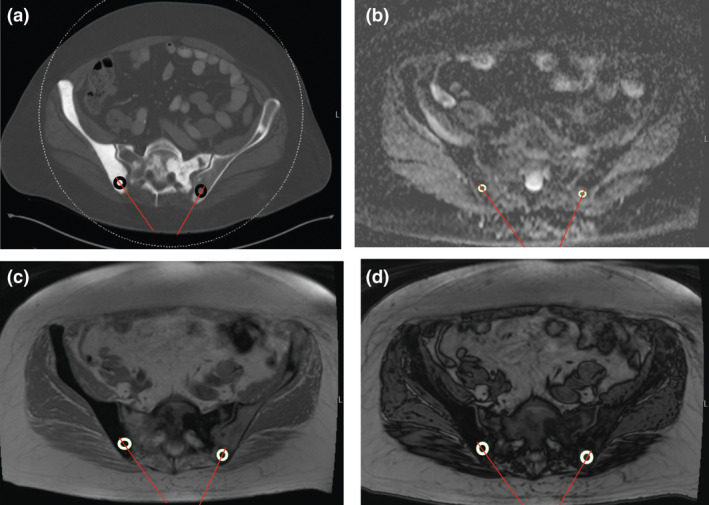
(A) Measurement of mean CT attenuation of lesions in the right and left posterior ilium in a 75‐year‐old man with suspected castrate‐resistant metastatic prostate cancer. The lesion in the right ilium had a CT attenuation of 1063 HU. The lesion in the left posterior ilium had a CT attenuation of 387 HU. Red lines show the biopsy needle paths. (B) Measurement of the signal intensity of lesions in the right and left posterior ilium on ADC MRI sequence in a 75‐year‐old man with suspected castrate‐resistant metastatic prostate cancer. The lesion in the right ilium had a mean ADC of 175 mm^2^/s and the lesion in the left posterior ilium had a mean ADC of 921 mm^2^/s. Red lines show the biopsy needle paths. (C) Measurement of the signal intensity of lesions in the right and left posterior ilium on in‐phase MRI in a 75‐year‐old man with suspected castrate‐resistant metastatic prostate cancer. The lesion in the right ilium had a mean signal intensity of 9.86 and the lesion in the left posterior ilium had a mean signal intensity of 41.46. Red lines show the biopsy needle paths. (D) Measurement of the signal intensity of lesions in the right and left posterior ilium on out‐of‐phase MRI sequence in a 75‐year‐old man with suspected castrate‐resistant metastatic prostate cancer. The lesion in the right ilium had a mean signal intensity of 6.42 (SIR 0.65) and the lesion in the left posterior ilium had a mean signal intensity of 50.59 (SIR 1.22). Red lines show the biopsy needle paths
